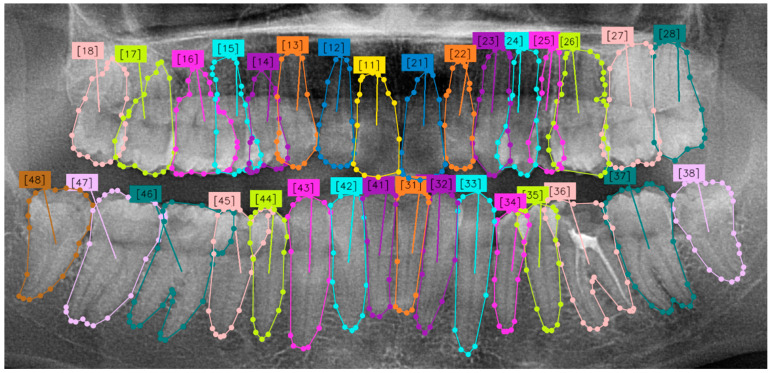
A panoramic radiograph annotated by the expert dentist according to the FDI numbering system.
